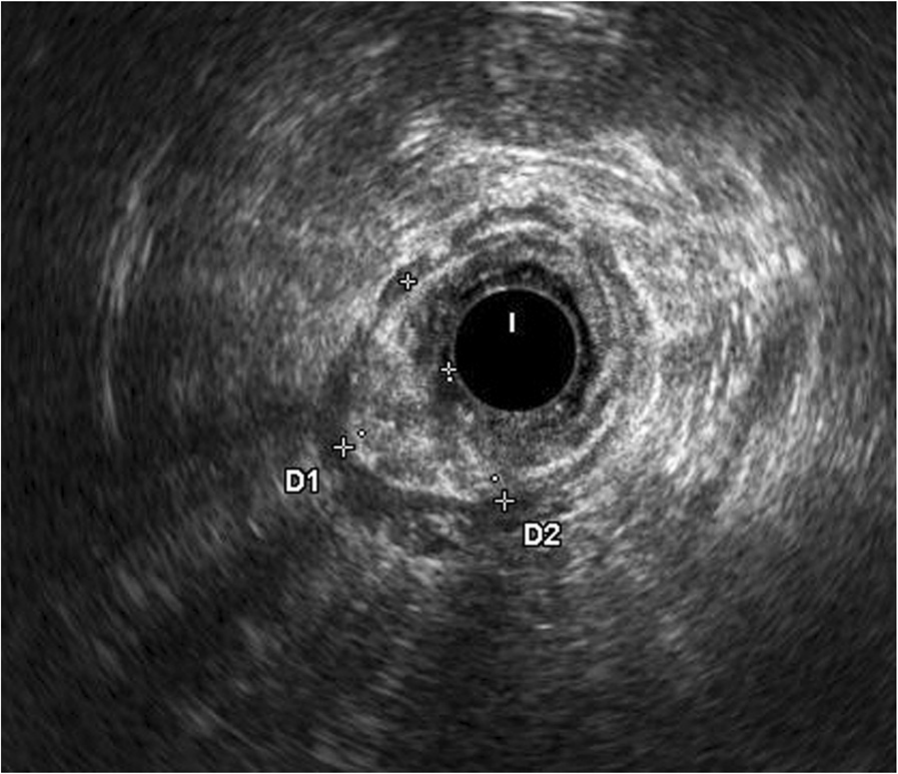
Endoanal ultrasound (US) showing the tumor invasion of the anal canal. Endoanal US allowed completion of the staging and showed the degree of infiltration thus staging the tumor as uT2.
